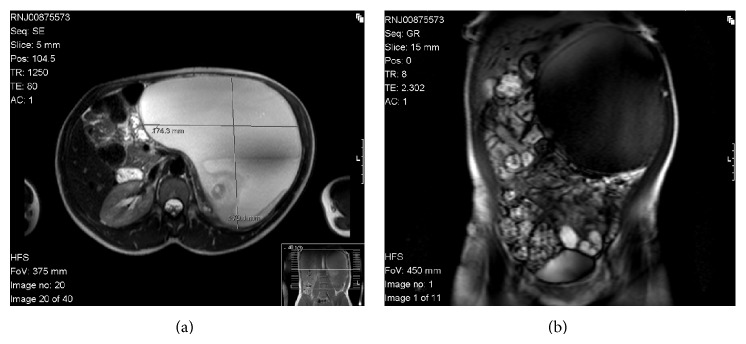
MRI images of the giant left retroperitoneal teratoma: (a) cross-sectional view and (b) coronal view.
